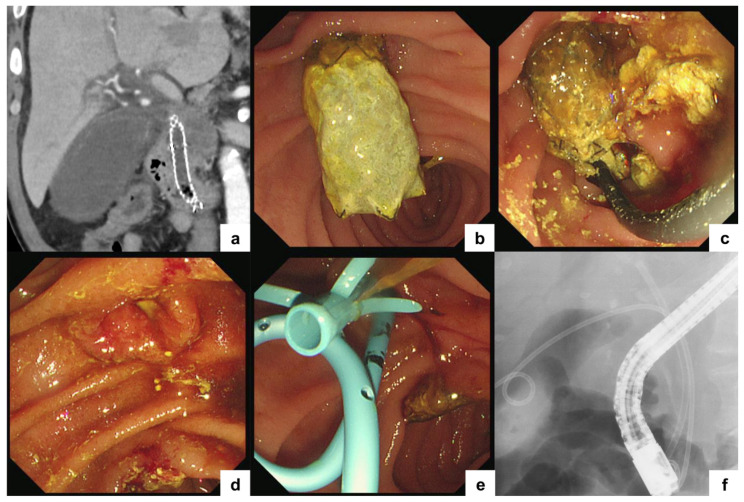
Endoscopic removal of a multi-hole self-expandable metallic stent (MH-SEMS). A patient with malignant distal biliary obstruction due to pancreatic cancer underwent MH-SEMS placement. (a) Computed tomography image obtained one month after stent placement demonstrating acute cholecystitis. Because of a bleeding tendency, percutaneous transhepatic biliary drainage and endoscopic ultrasound-guided gallbladder drainage were contraindicated. (b) Endoscopic view of the indwelling MH-SEMS. (c,d) Endoscopic images demonstrating MH-SEMS removal. The stent was grasped with a snare and successfully retrieved without any adverse events. (e,f) Plastic stents were subsequently placed in the bile duct and gallbladder.
